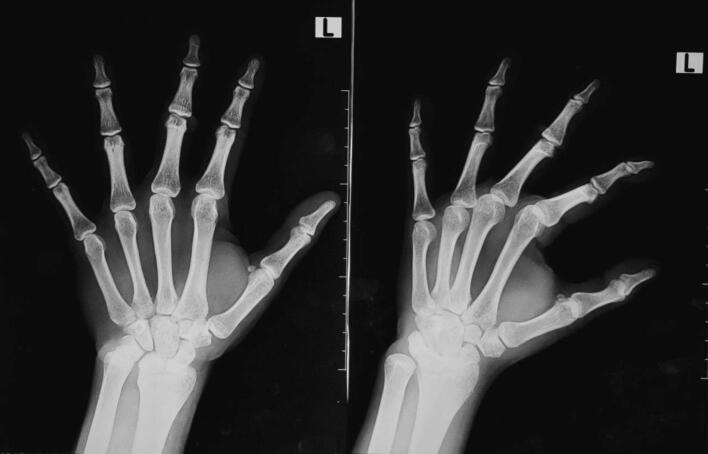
Pre-operative plain AP/Oblique X-ray of the left hand.
